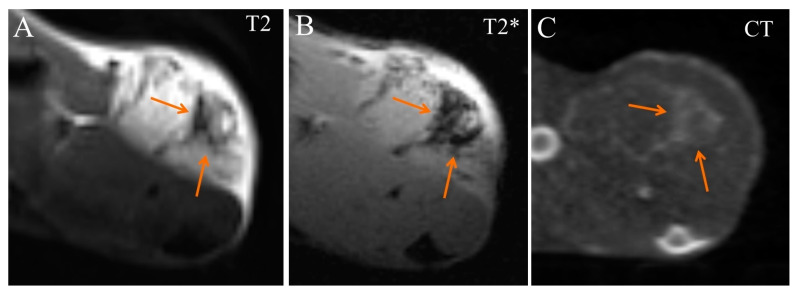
Transversal images of Ca755 mammary carcinoma. (A) T2 MRI 50 min post injection of Fe_3_O_4_@Au; (B) T2* MRI 50 min post injection of Fe_3_O_4_@Au; (C) CT image 65 min post injection of Fe_3_O_4_@Au. Arrows mark the enhanced area of the tumor.
